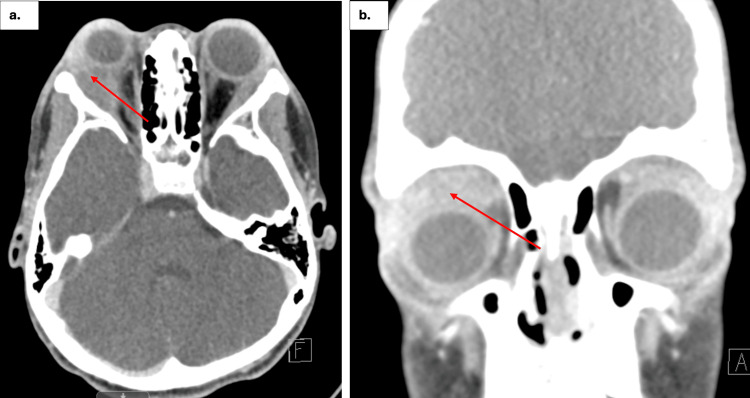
Latest CT scan of the orbit/sella/ear (with contrast) a. The axial CT scan (left) shows asymmetric proptosis with greater enlargement of the right lacrimal gland compared to the left. The right lacrimal gland appears larger compared to previous imaging (Figure 1), resulting in an increased mass effect on the right globe. The enlarged right lacrimal gland is indicated by a red arrow. b. The coronal CT scan (right) also demonstrates the enlargement of the right lacrimal gland. In this view, the enlarged gland is highlighted with a red arrow. In both the axial and coronal planes, the red arrows point out the enlarged right lacrimal gland, with no evidence of an abscess.
